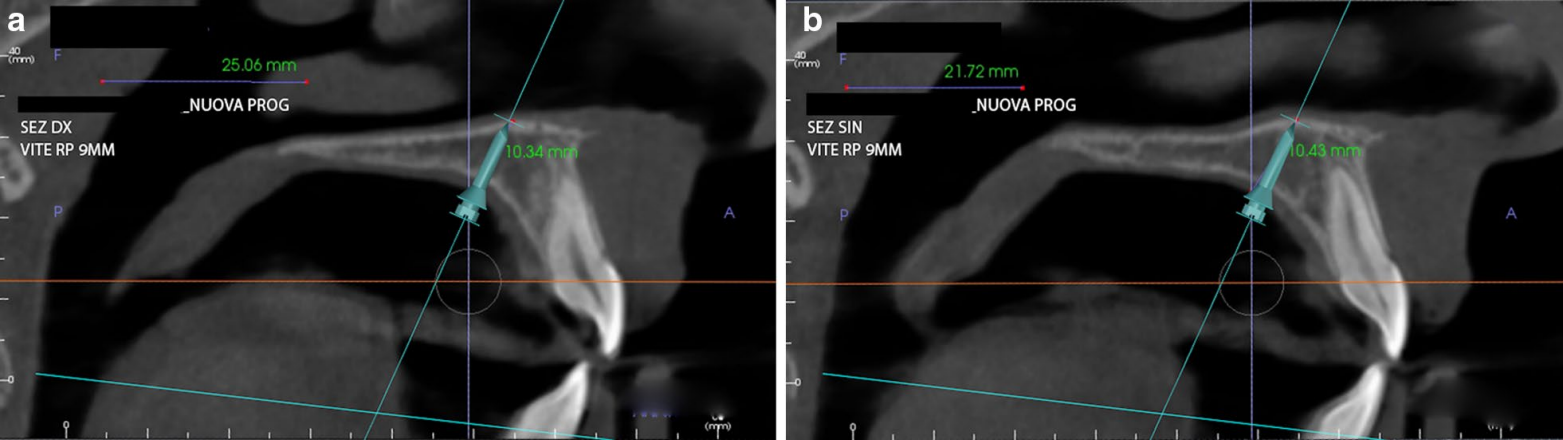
a, b Planning of miniscrews insertion on CBCT
